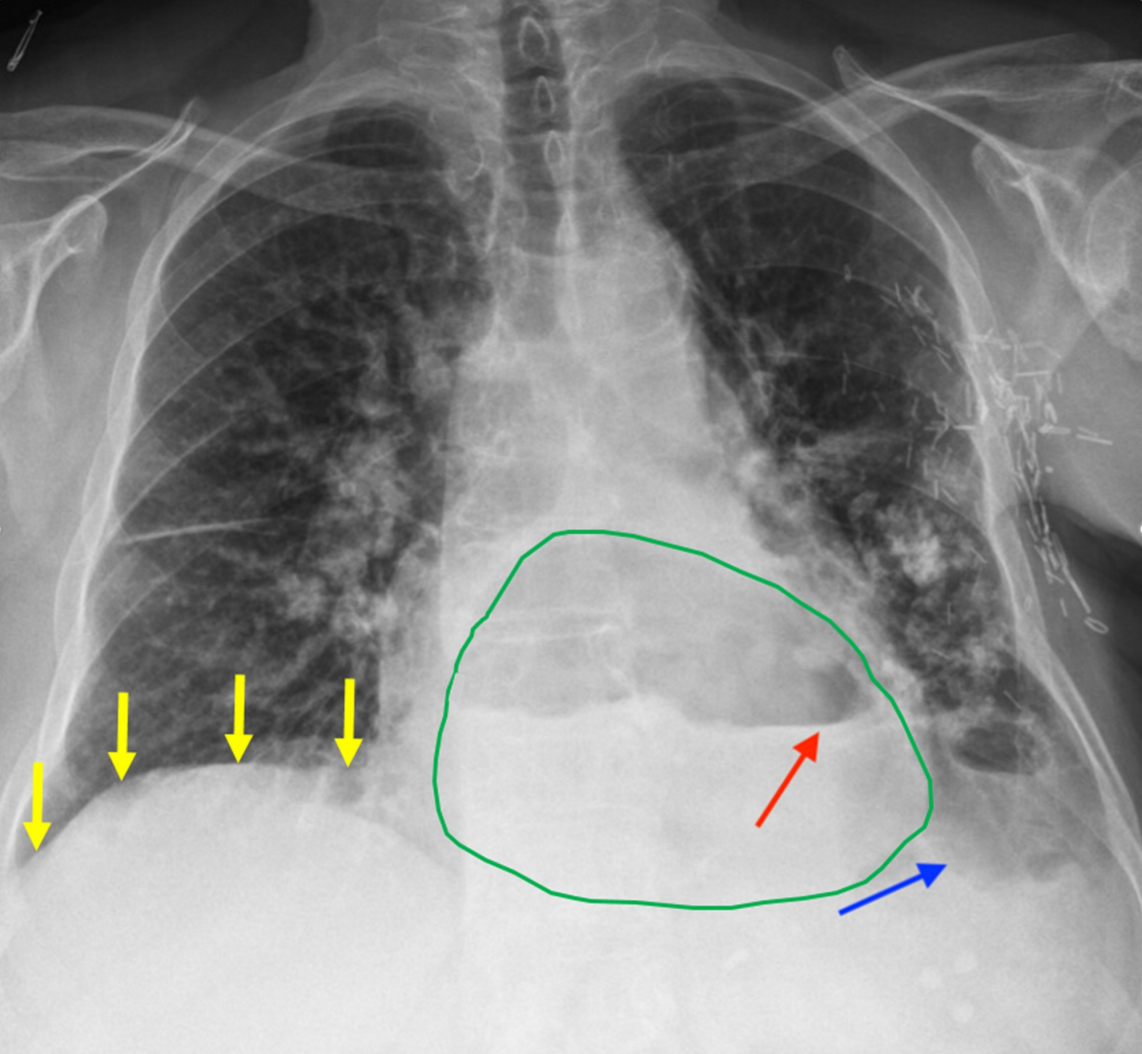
Chest radiograph revealing a massive herniation of intra-abdominal contents Abdominal contents herniating (green outline) above the level of the diaphragm (yellow arrows) into the thorax are observed. The air-fluid level of the stomach (red arrow) is identified above the level of the diaphragm. A small pleural effusion is seen in the lower lobe of the left lung (blue arrow).
